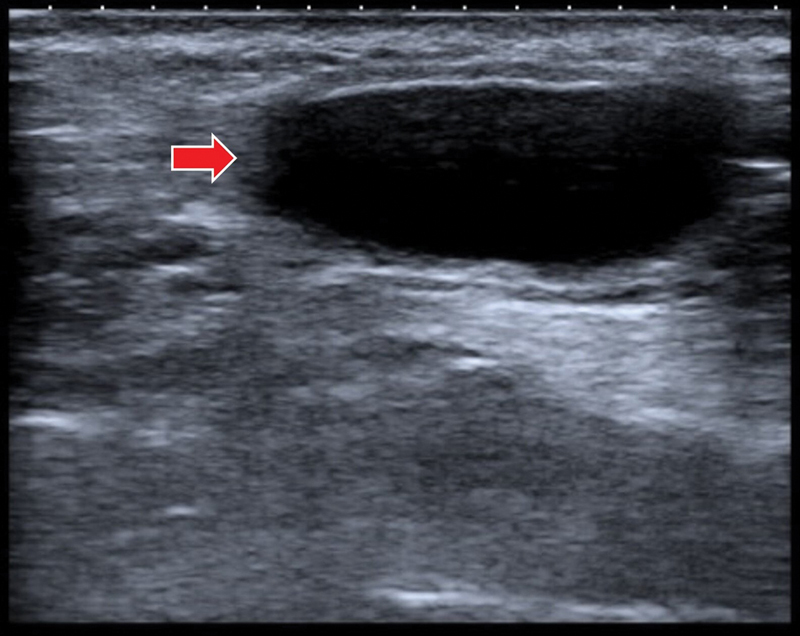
Breast ultrasound showing a 7 cm × 5 cm cystic image with thin walls (red arrow).
